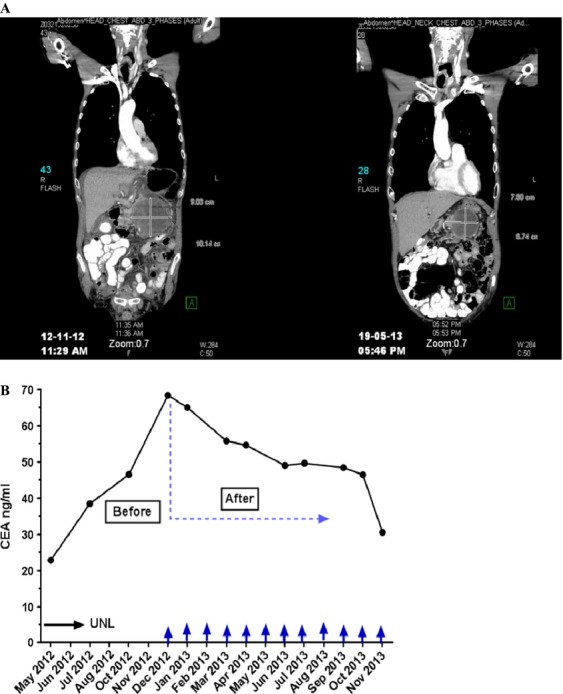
Antitumor activity of PL-MLP. (A) CT image showing response to treatment in a melanoma patient with disappearance of ascites and volume shrinkage of abdominal tumor mass (>30%) for 6 months. Reported as Stable Disease (as two small lesions in lung and forehead—not shown—increased in size). (B) Plot showing CEA decreases in a colon cancer patient receiving 12 cycles of PL-MLP. From start of PL-MLP, the CEA level dropped gradually by >2-fold. Stable Disease in CT-scan for 12+ months.
